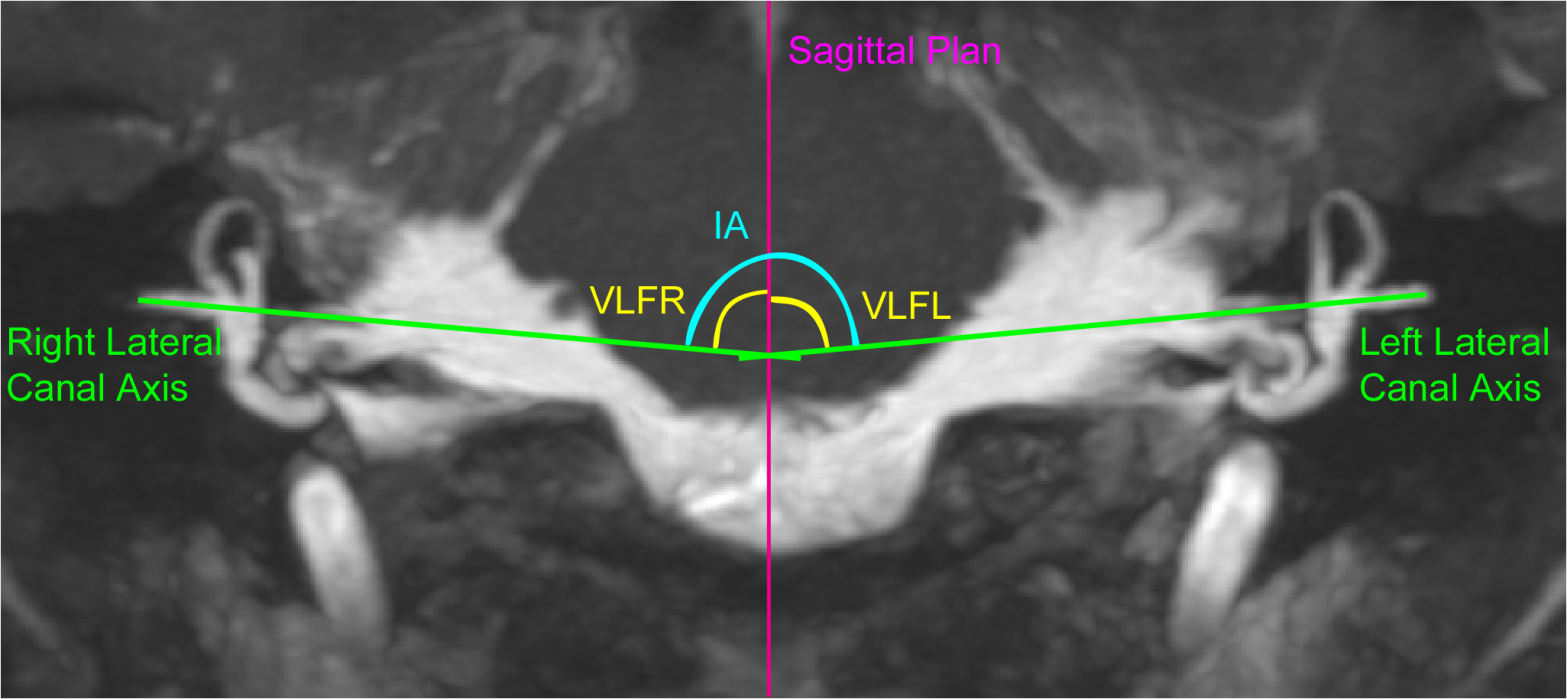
Measurement of the lateral semicircular canal in the frontal plane. MRI image in the frontal plane showing the “*Intercanal Angle*”(IA) formed by the axis of the right and left lateral canals; the “*Vertical Lateral canal Frontal angle Right*”(VLFR) formed between the right lateral SCC and the vertical axis (defined by the sagittal plane); and the” *Vertical Lateral canal Frontal angle Left” (*VLFL) formed between the left lateral SCC and the vertical axis.
